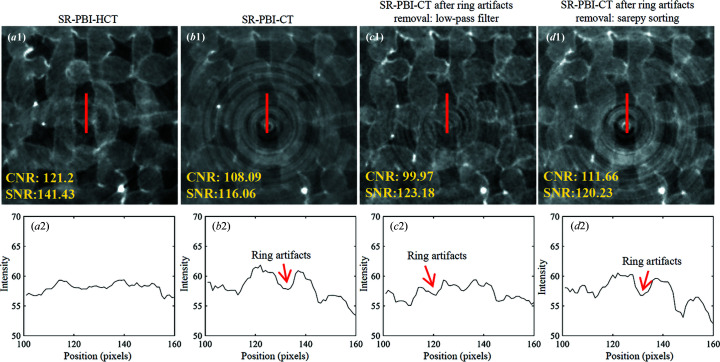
Comparison of SR-PBI-HCT and SR-PBI-CT (gray scale: 0–255); (*a*1) SR-PBI-HCT image, (*b*1) SR-PBI-CT image, (*c*1) SR-PBI-CT image with low-pass Fourier filtering and (*d*1) SR-PBI-CT image with Sarepy sorting. (*a*2)–(*d*2) corresponding gray value profiles of ROIs at red line positions.
